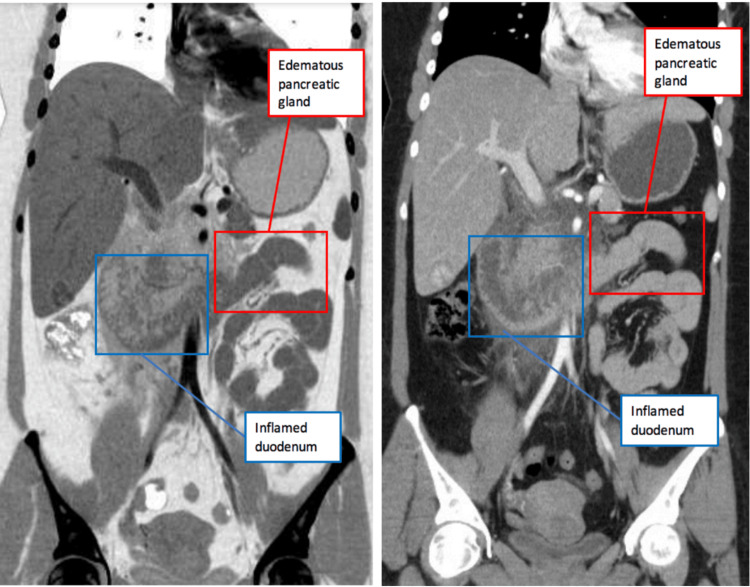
CT Scan of Abdomen in the coronal plane showed findings consistent with uncomplicated pancreatitis and inflammatory changes in the duodenum most likely as a secondary reaction to the adjacent acute pancreatitis
